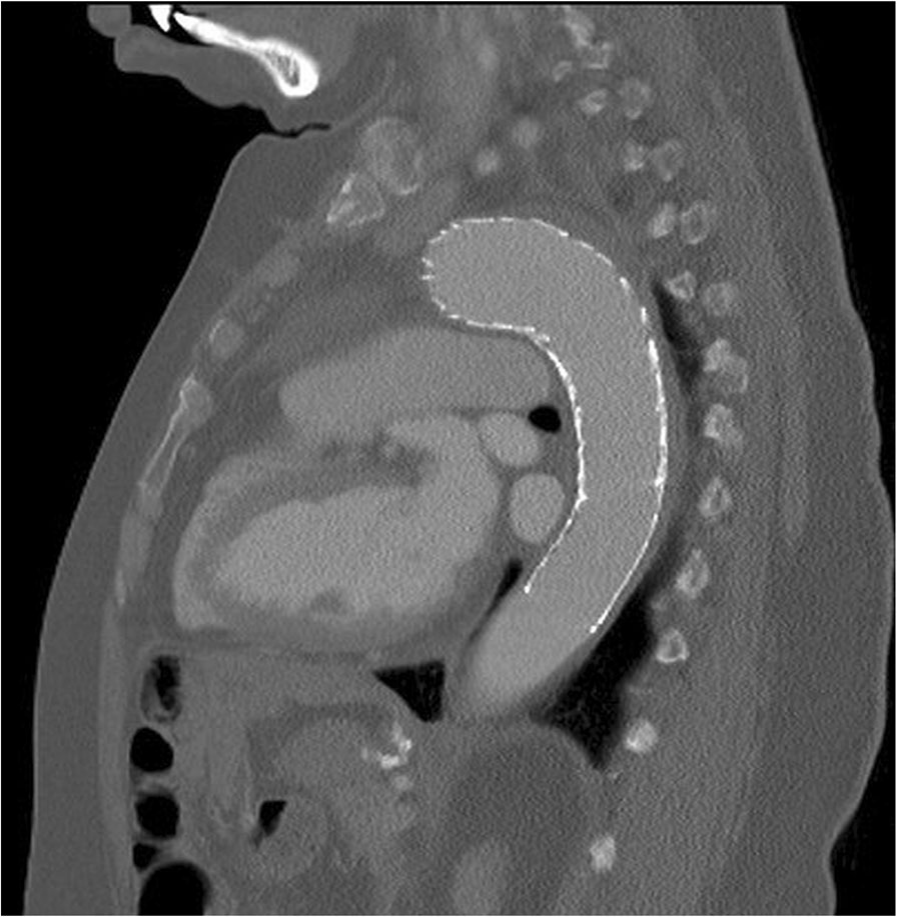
Follow up CTA (sagittal view) one month after TEVAR showing widely patent aortic stent graft without any evidence of extravasation or endoleak
